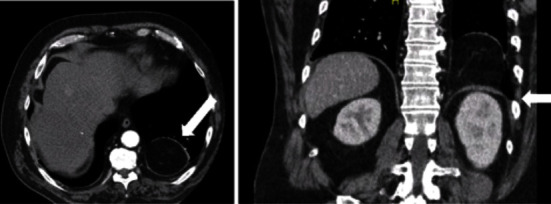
Axial and coronal slice CT scans depicting BH.
